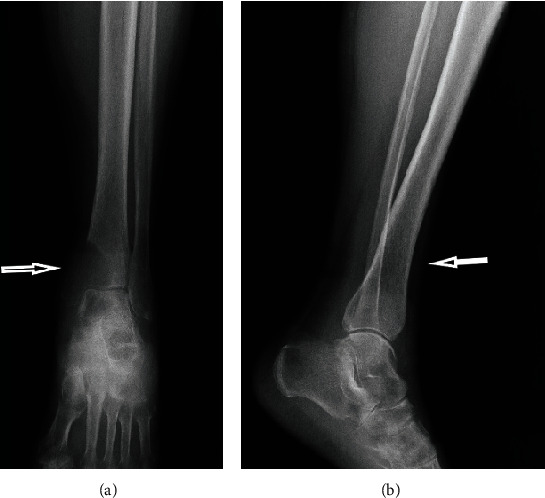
X-ray anteroposterior/lateral view of the leg showing destruction of the distal tibia.
